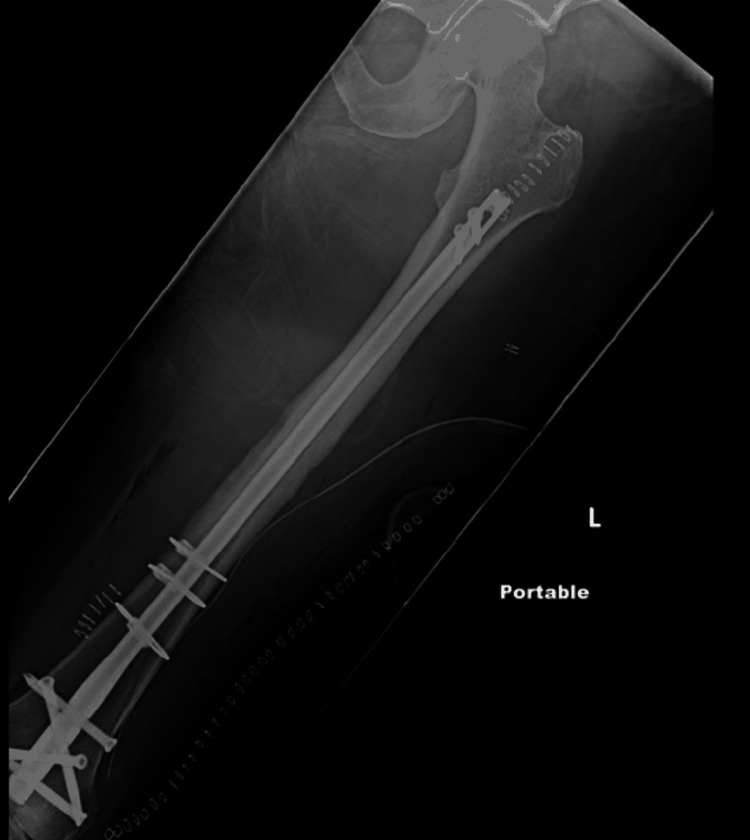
Postoperative open reduction and internal fixation (ORIF) x-ray showing good fixation
